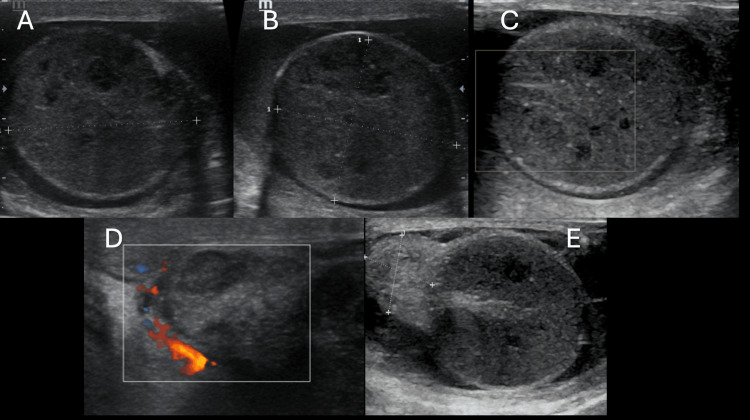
Testicular Torsion Figures 1A, 1B shows left side testicular enlargement, which appears edematous and heterogenous in architecture with multiple hypo-echoic spaces within. Figure 1C shows no evidence of vascularity on color Doppler. Figure 1D shows twisting of the spermatic cord noted above the level of testis. Figure 1E shows an enlarged and heterogenous epididymis.
